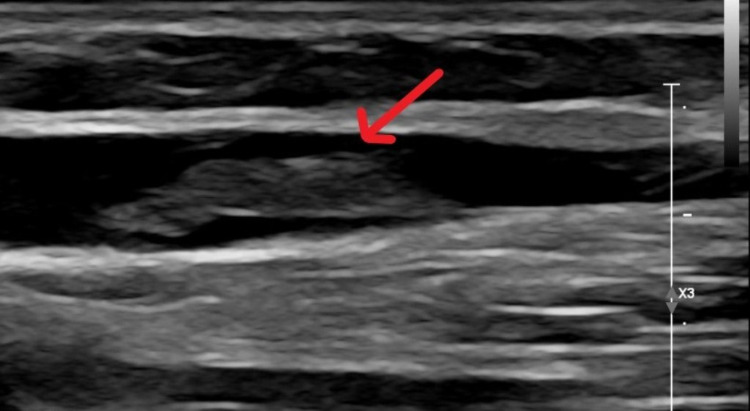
Ultrasound of right upper arm showing thrombus in basilic vein.
